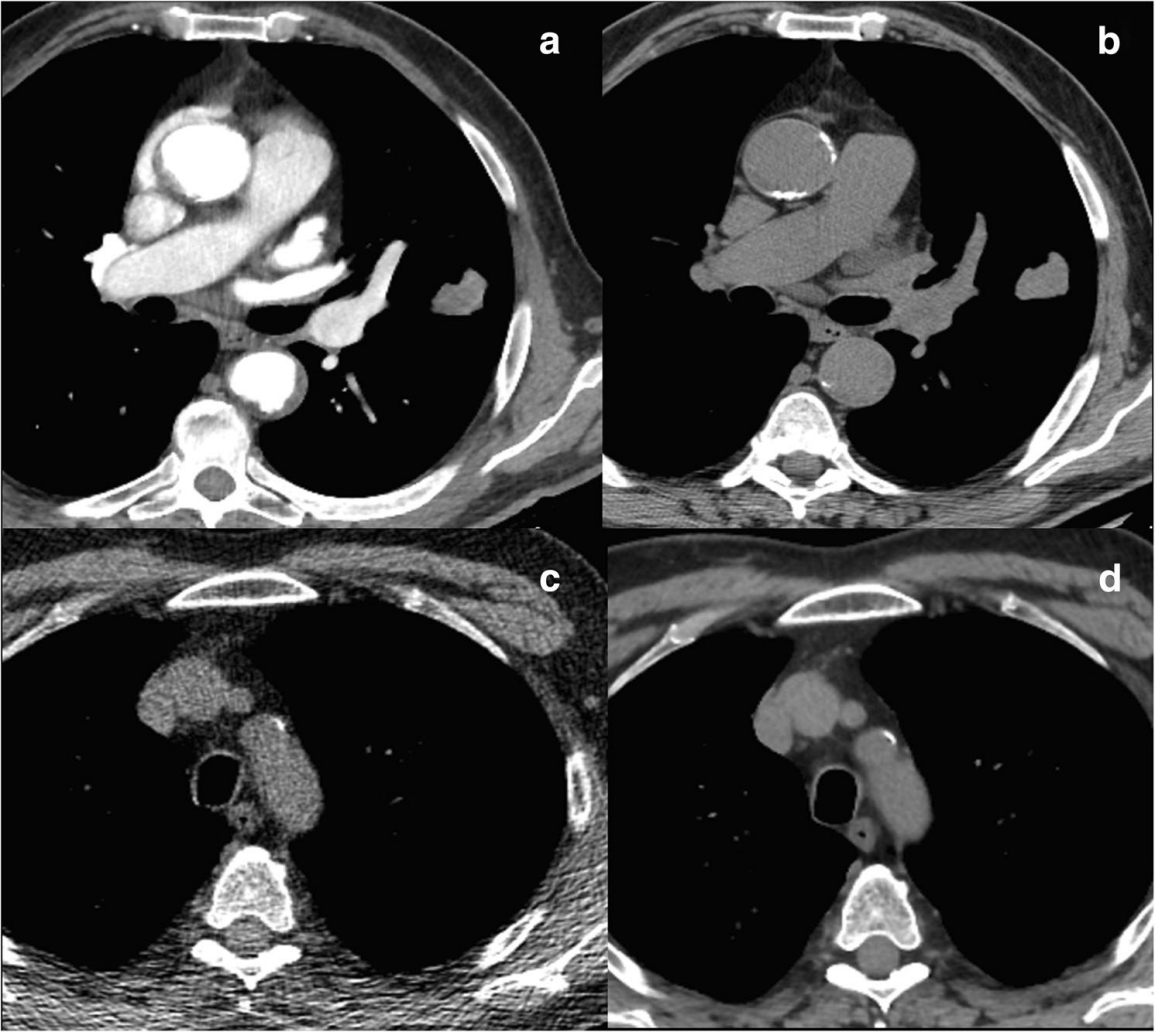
Axial computed tomography images showing differences in the same acquisition plane between a contrast-enhanced (a) and an unenhanced image (b), as well as for different radiation doses, lower in (c), and higher in (d)
